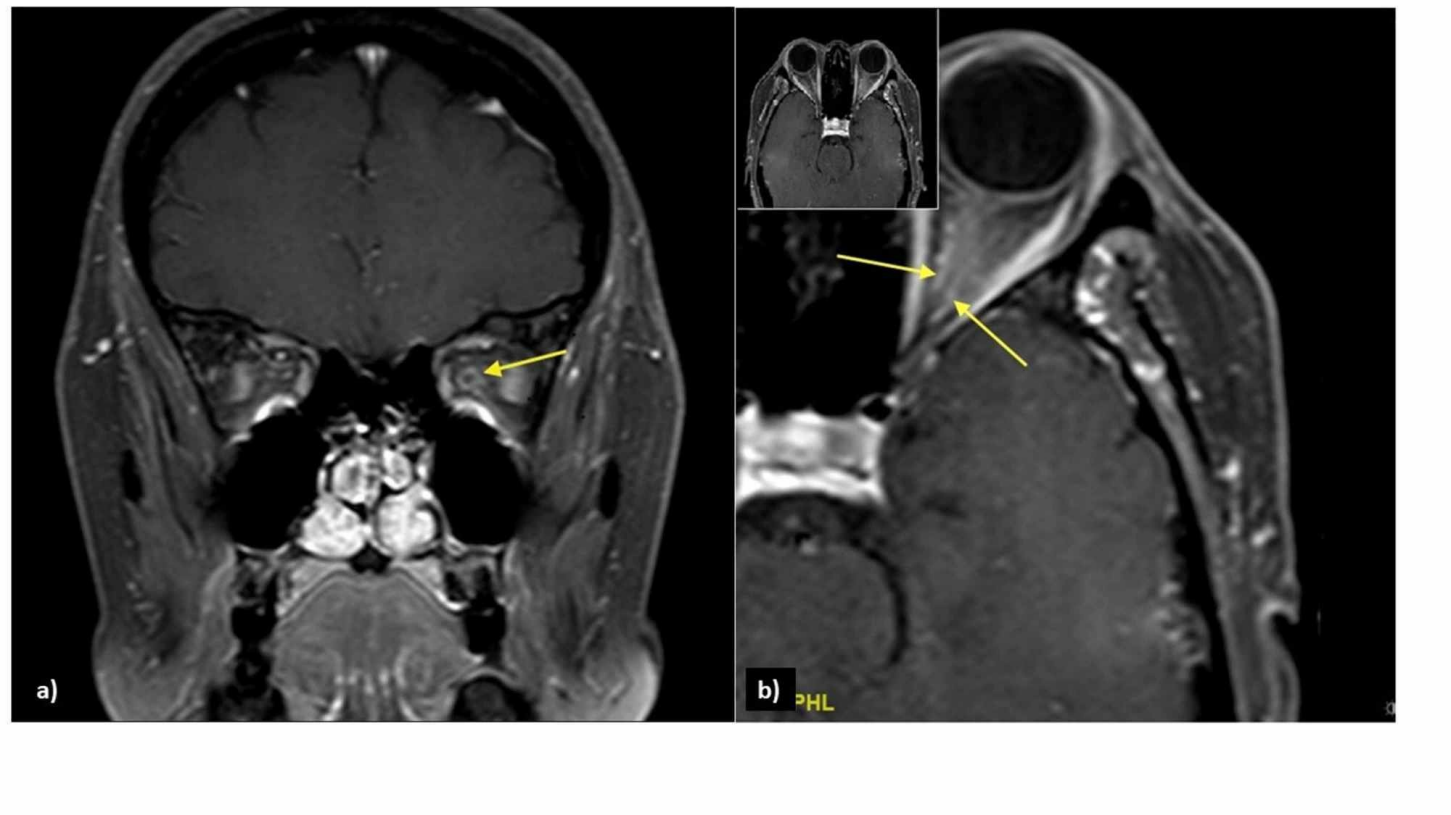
Post-contrast T1-weighted image with fat suppression shows: a) doughnut sign on coronal view (arrow) and b) tram-track sign on axial view (arrows)
